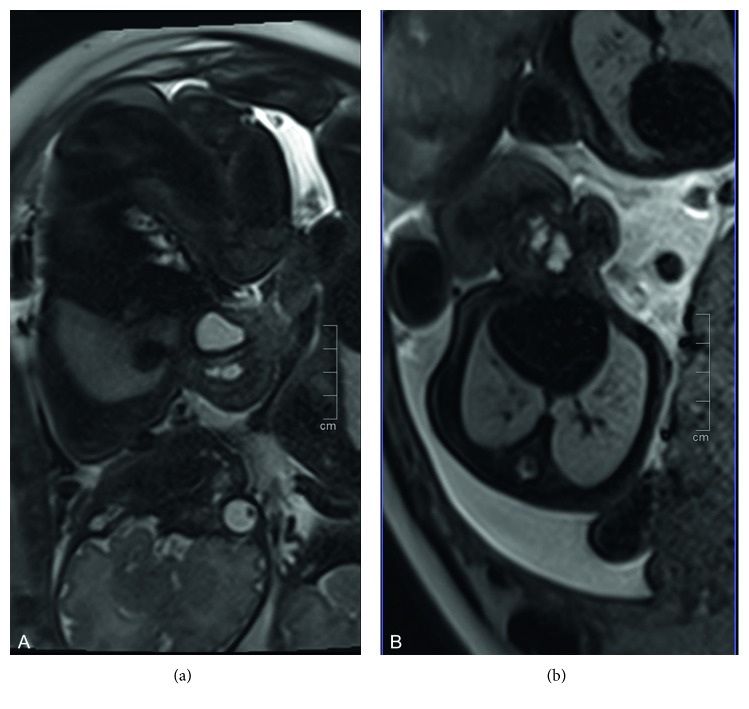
Preterm MRI depicting a ventral corpus to the autosite on the right in sagittal view (a). Same situation in cross-sectional plane (b).
